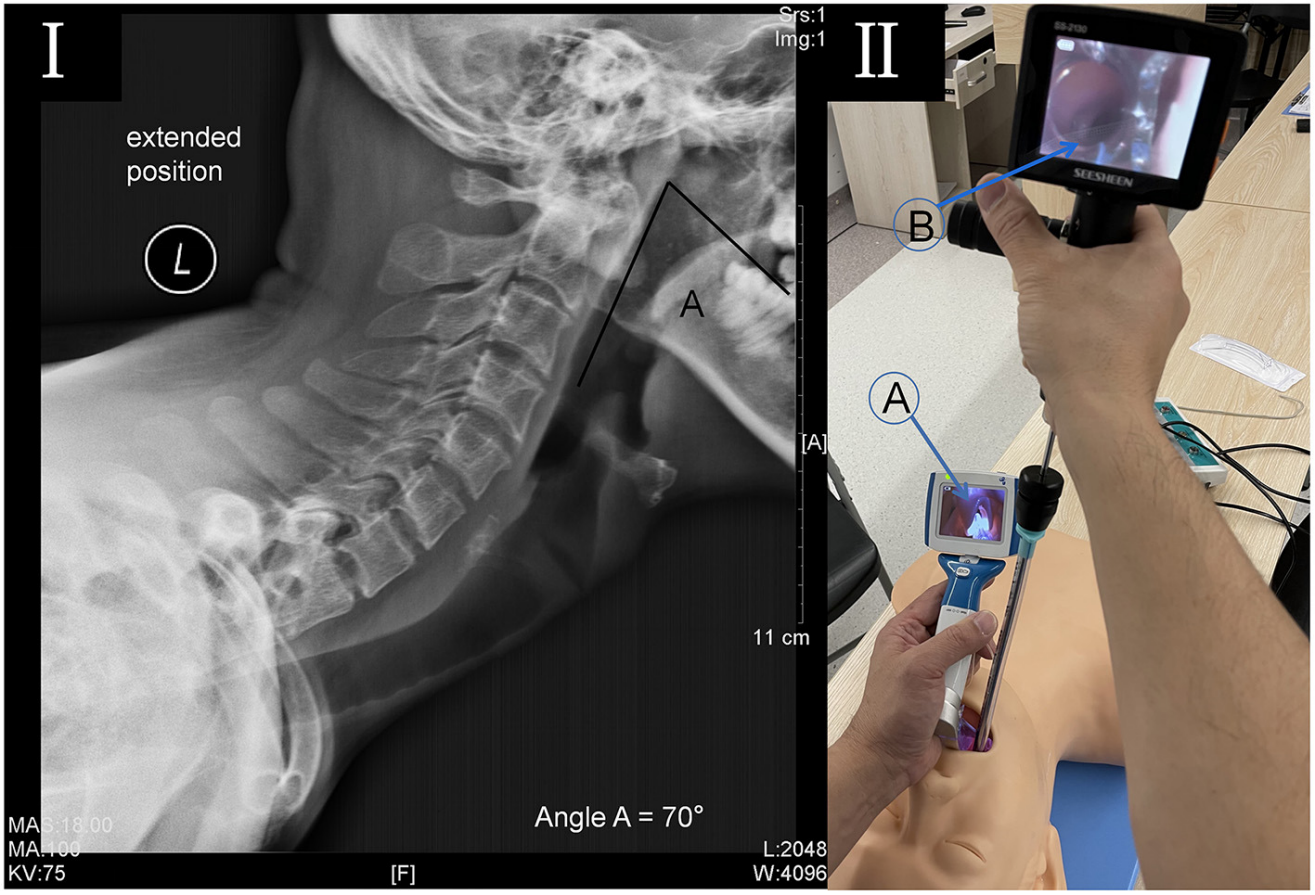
(I) Lateral radiograph of the cervical spine in full extension. The photograph demonstrates that hyperostosis was seen at the cervical vertebral body margin, and the oropharyngeal axis (angle a) was 70°. (II) A double visualized intubation on a mannequin. (A) The video laryngoscope opens the oropharynx to show the epiglottis; (B) The lighted stylet uncovers the epiglottis to expose the glottis and guide the tracheal tube into the trachea.
